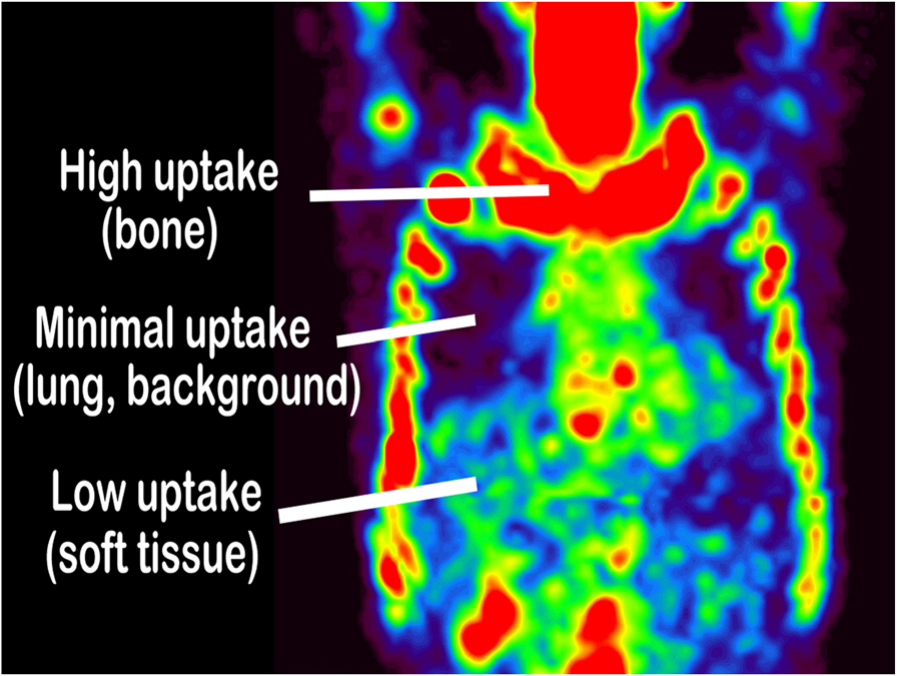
Segmentation of bone SPECT data into high, low, and minimal uptake areas for attenuation correction
